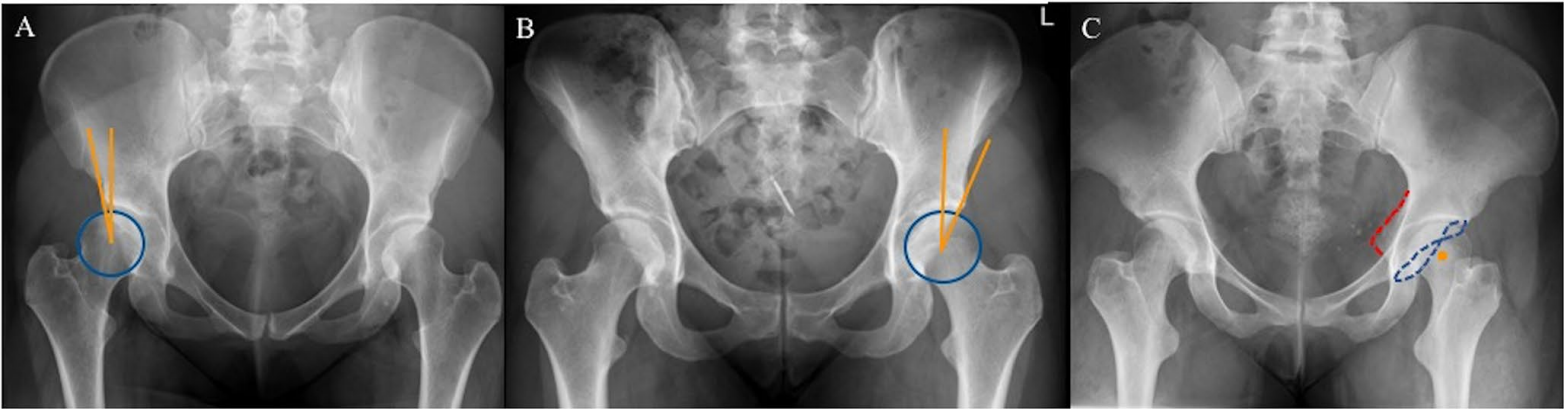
Representative anteroposterior pelvic radiographs of (A) hip dysplasia – LCEA < 18° - orange line (B) borderline hip dysplasia LCEA 18° − 25° - orange line, and (C) acetabular retroversion - red sciatic spine sign, blue crossing-over sign, orange posterior wall sign. LCEA lateral center-edge angle
